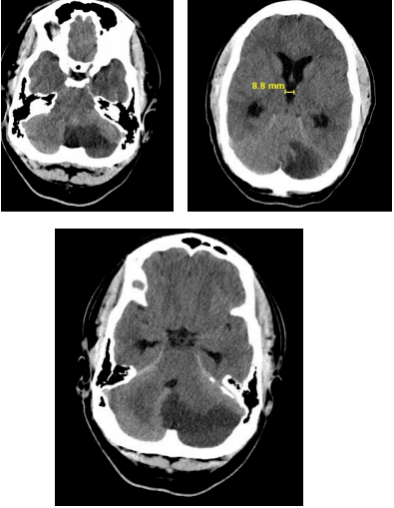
CT Demonstrating Progressed Dilation of Third and Lateral Ventricles.
